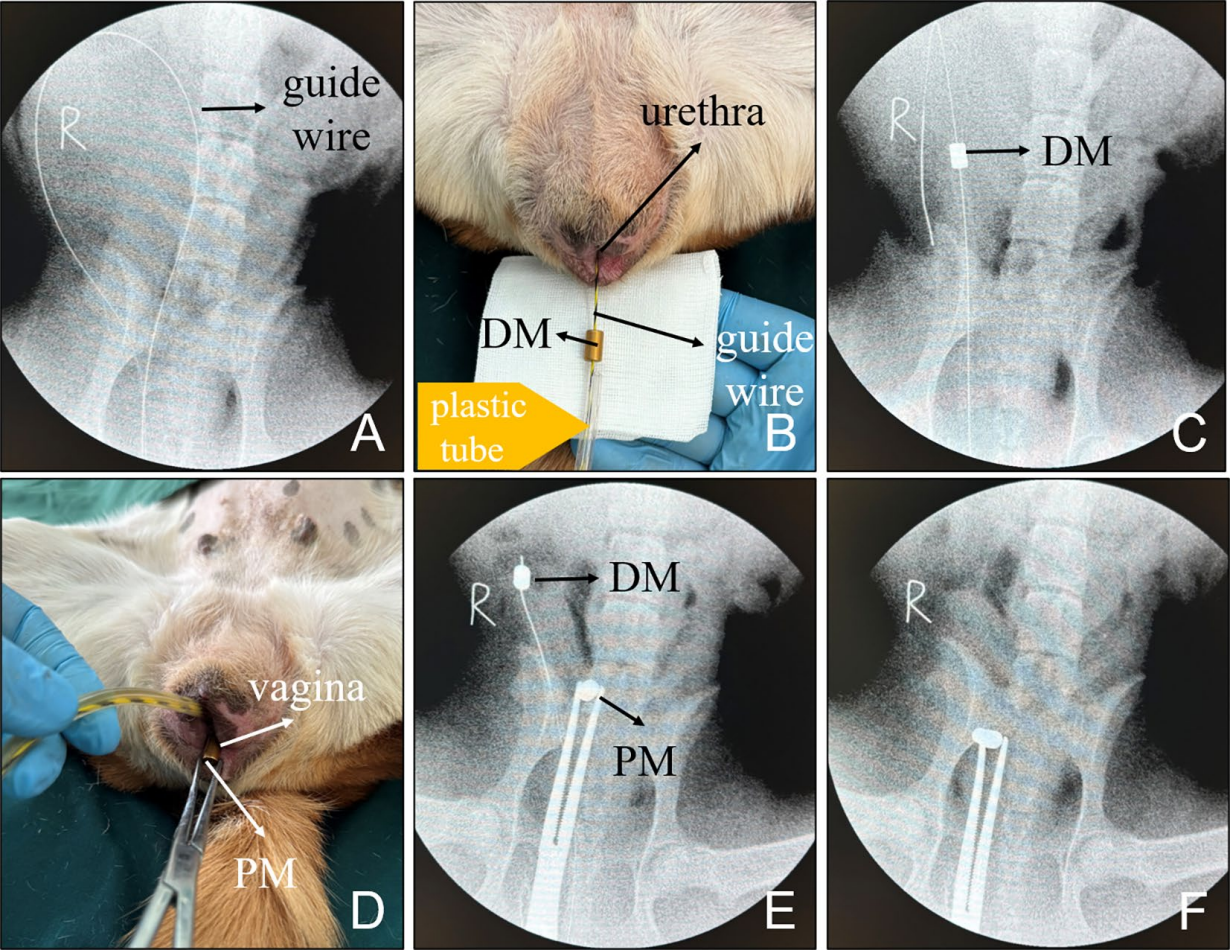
Representative images taken during the operation. (A) X-ray after the guidewire entered the bladder. (B) The DM followed by the plastic tube is inserted through the tail end of the guide wire. (C) The plastic catheter pushes the DM into the bladder. (D) The PM is inserted vaginally using a vascular clamp. (E) The position of the magnets is observed under X-ray. (F) The magnet position is adjusted such that the DM and PM are attracted to each other.
